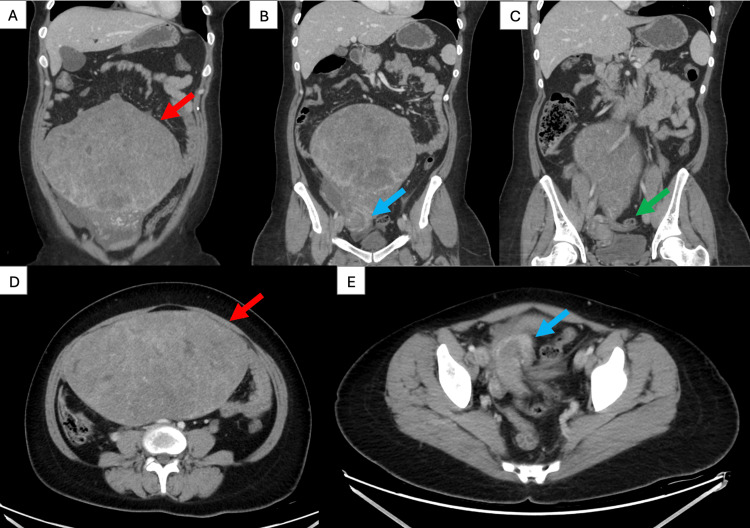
Computed tomography (CT) Contrast-enhanced cross-sectional imaging of the abdomen and pelvis showing a large superficial subserosal uterine mass, originating from the fundus and causing uterine torsion. The left adnexa is seen on the right side of the pelvis, with crossing of the left gonadal vessels through the torted uterine pedicle to the right side. (A, D) massive uterine fibroid, (B, E) uterine torsion caused by the fibroid, (C) left gonadal vessels crossing to the right side.
